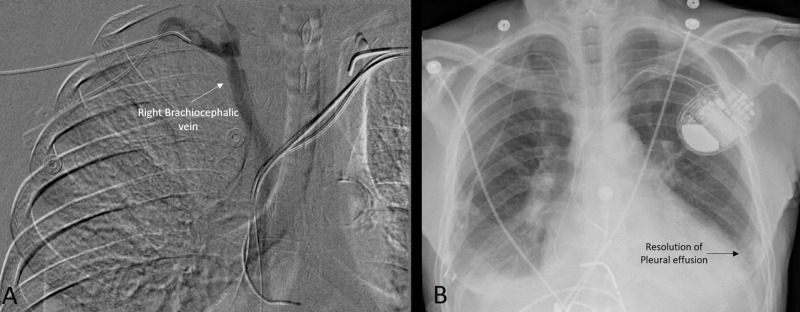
Imaging after the intervention The flow of contrast through the right brachiocephalic vein and superior vena cava can be seen (A) post balloon angioplasty. This led to near complete resolution of the left-sided pleural effusion as seen on chest x-ray (B).
